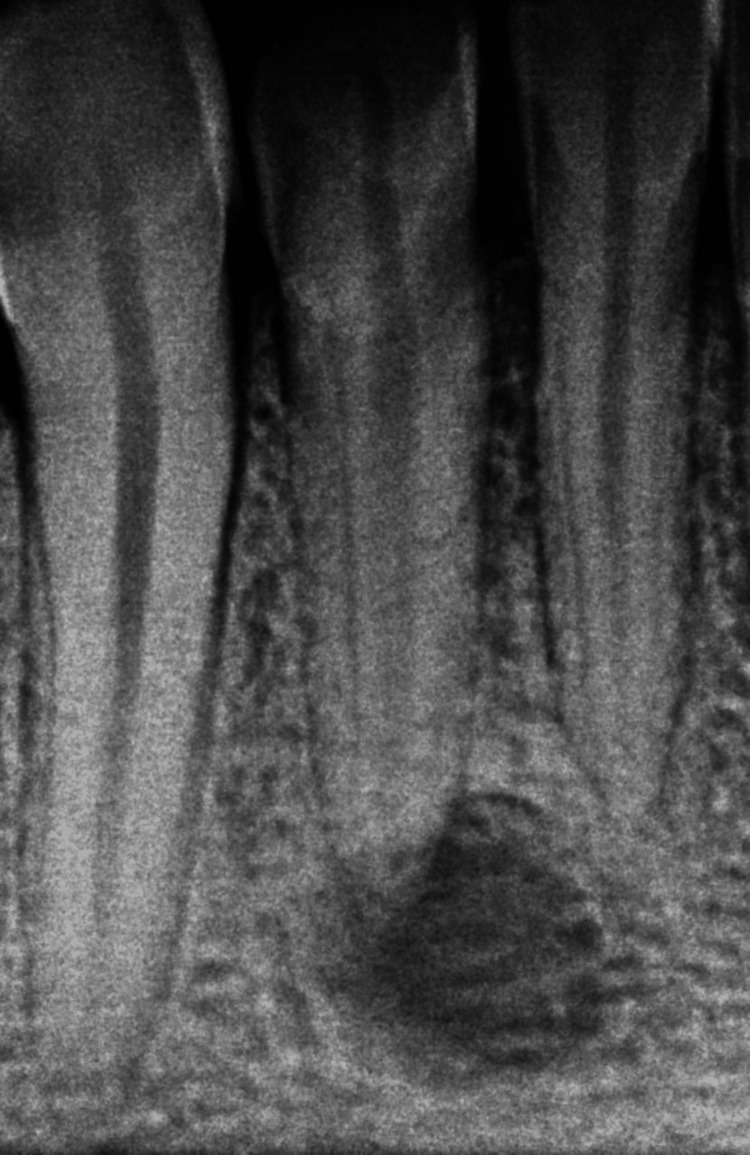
Preoperative intraoral periapical radiograph
